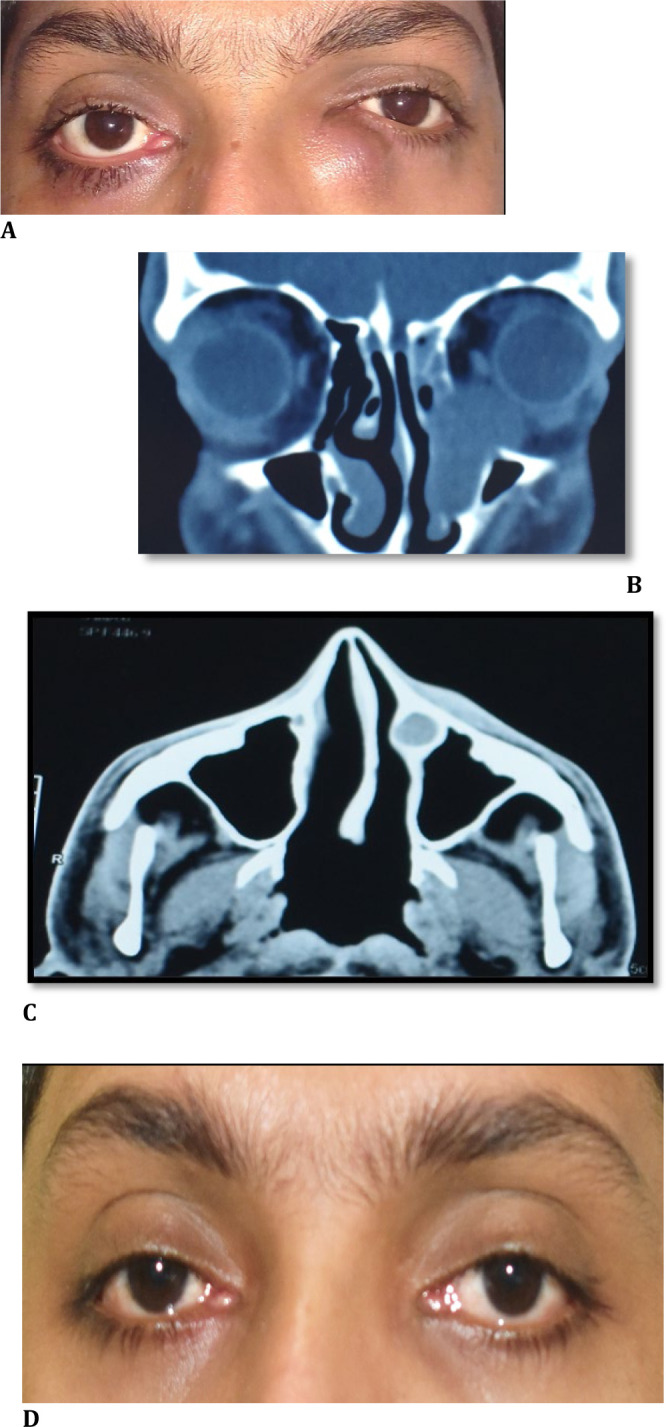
A. Clinical picture showing medial canthal swelling and superolateral dystopia in the left eye; B, C. CT scan displaying the expansion of the cystic lacrimal sac into the orbit with a dilated nasolacrimal duct; D. Patient at one-year follow-up visit
